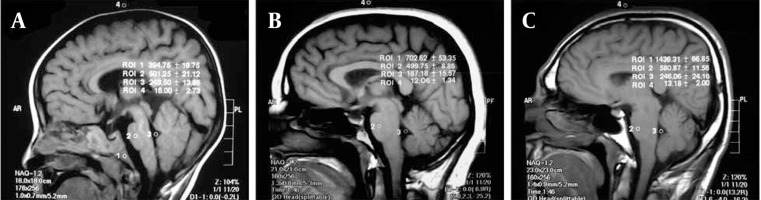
Quantitative assessment of clivus bone marrow signal intensity based measurements on MRI. A, In a 2-year-old boy; B, In a 42-year-old woman; C, In a 34-year-old man (1. Clivus, 2. Pons, 3. CSF, 4. Air)
